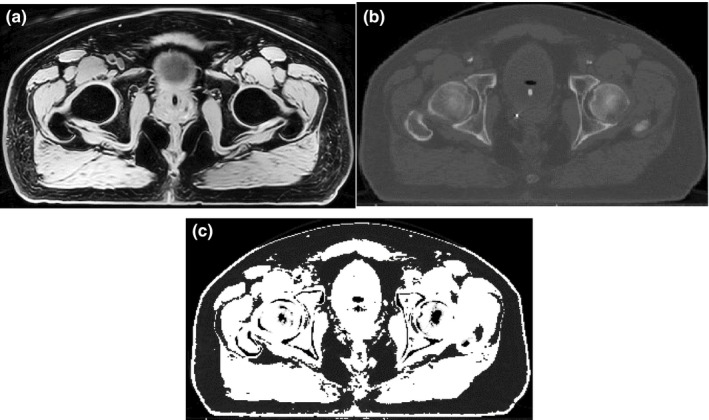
Example of a standardized water‐only image (a), planning CT (b), and fat‐ and bone‐suppressed CT (c). Bone and fat suppression was performed to improve the similarity in image contrast between the CT and water‐only MR images, thereby facilitating the deformation of the CT to the magnetic resonance imaging.
